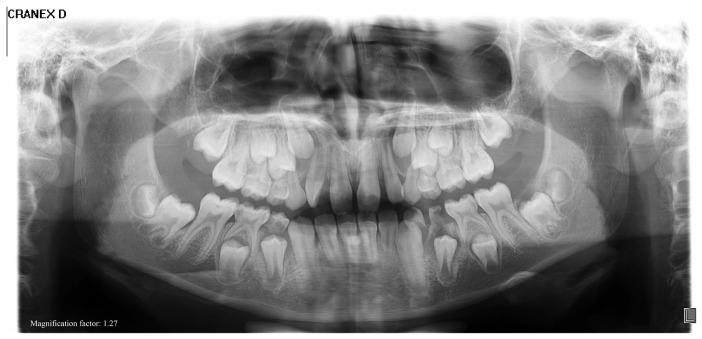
Initial panoramic radiograph showing bilateral elongation of the coronoid processes. The structural abnormality is evident on both sides and correlates clinically with the patient’s restricted mouth opening.
